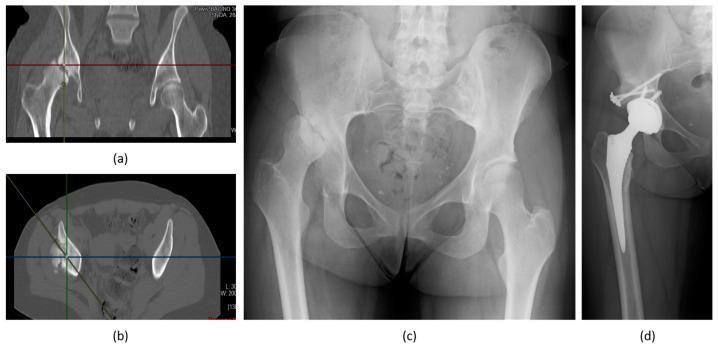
Female patient, 53 years old, with HIV, treated for acetabulum and femoral head AVN (avascular necrosis) with bone graft and total hip arthroplasty (THA). CT coronal (a) and axial (b) images, and X-rays ap view (c) showing the right femoral head and acetabulum AVN left untreated brought to a hip dislocation. Final treatment in the XR (d) showing bone grafting and THA. People living with HIV suffer from bone mineralization abnormalities, including osteoporosis and osteopenia; they can have AVN of the major joints.
